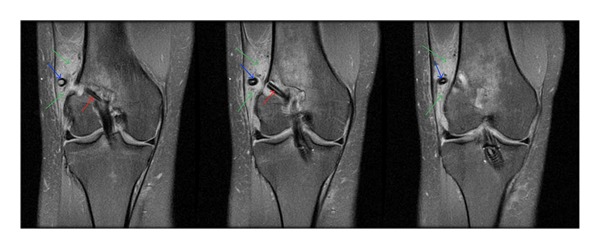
Coronal fat suppressed T2-weighted images demonstrate that the proximal portion of the pin has backed out of the distal femur and is found within the adjacent soft tissues where it is surrounded by edema (blue arrows) and a thickened iliotibial band (green arrows). The distal portion of the pin (red arrows) and ACL graft are intact.
